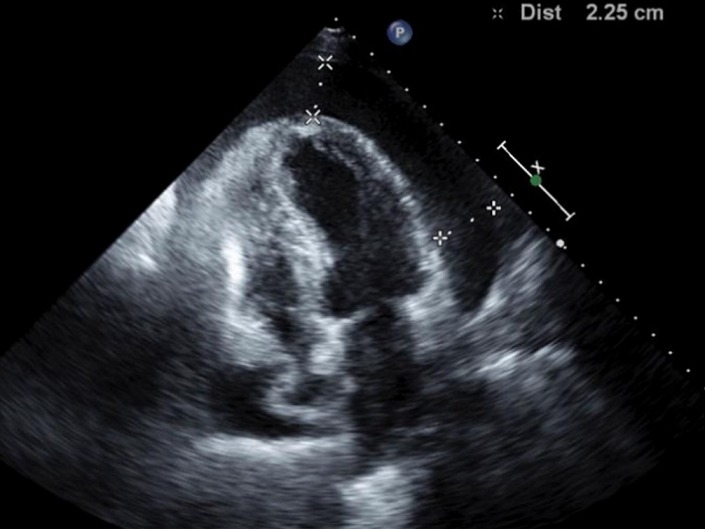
Echocardiographie transthoracique chez la patiente numéro 2: coupe apicale montrant un épanchement péricardique prédominant en regard des cavités gauches
